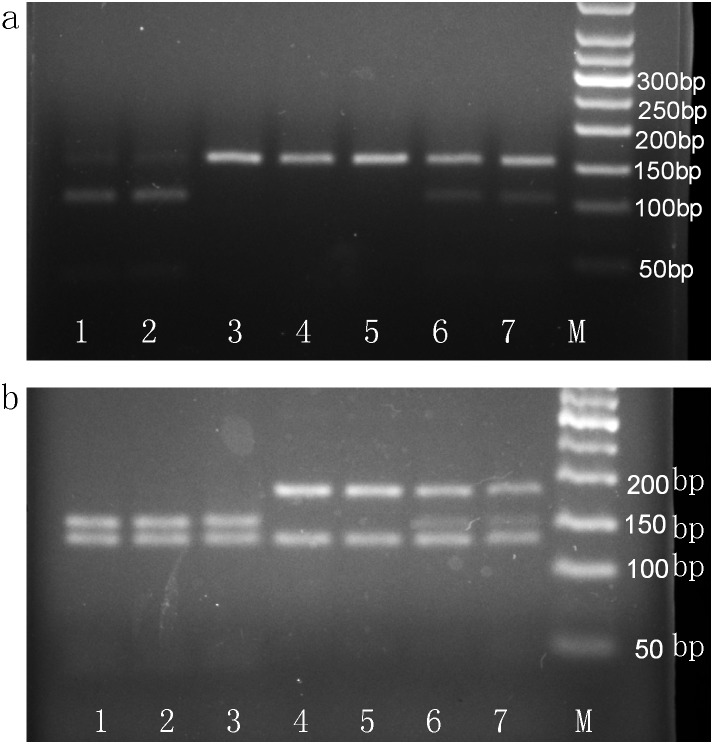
PCR-RFLP assay for analyzing the IL-4−589C/T and −33C/T polymorphisms of the IL-4 gene. PCR product was digested by restriction enzyme and visualized on a 2.5% agarose gel. a −589C/T polymorphism. Lanes 1 and 2 show CC genotype; lanes 3, 4 and 5 show TT genotype; lanes 6 and 7 show CT genotype. b −33C/T polymorphism. Lanes 1, 2 and 3 show TT genotype; lanes 4 and 5 show CC genotype; lanes 6 and 7 show CT genotype. M, marker.
